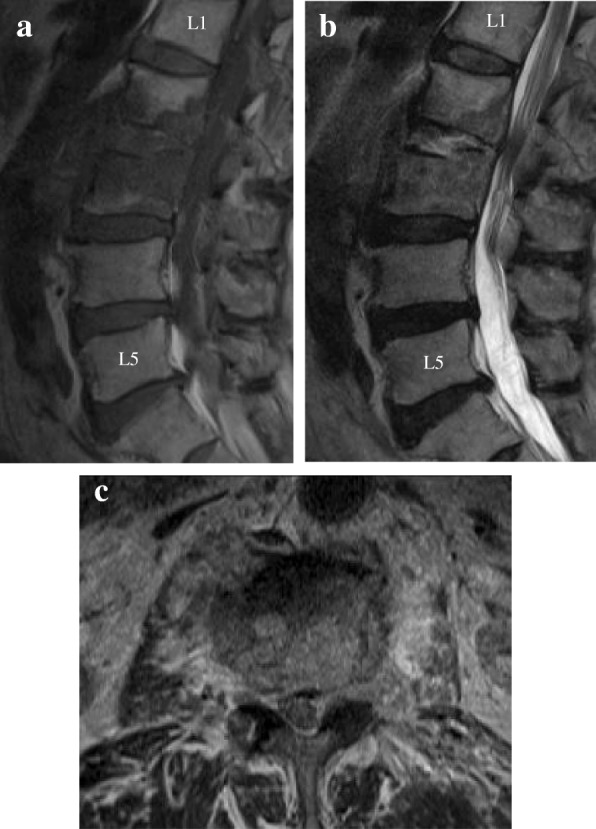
Magnetic resonance images of our patient. a. Sagittal T1-weighted images displaying a decreased signal in the L2/3 disc and the vertebral bodies. b. Sagittal T2-weighted images displaying an increased signal in the L2/3 disc and fluid collection in the anterior part of the vertebral bodies. c. Axial T2-weighted images displaying an increased signal around the posterior portion of the vertebral bodies, which extended into the left epidural space and reached the peripheral muscle tissue and the area near the aorta
